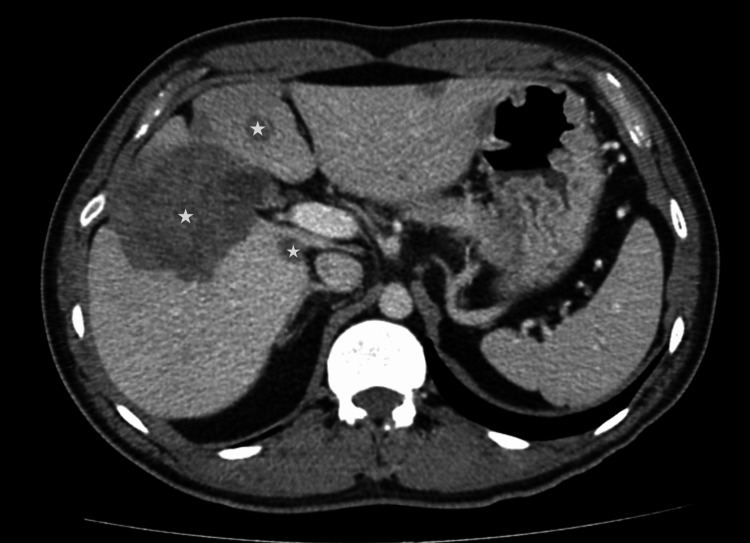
CT scan of the abdomen showing multiple hypoattenuating lesions throughout the liver ☆ indicates the hypoattenuating lesions.
